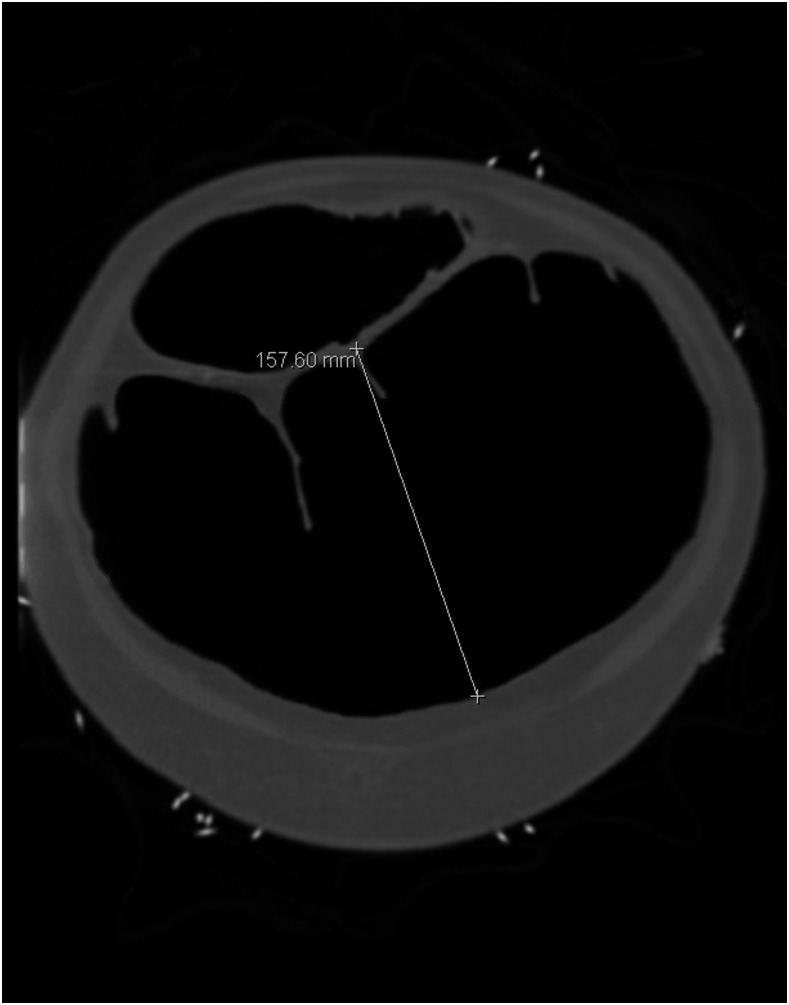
Computed tomographic study of the abdomen and pelvis in the coronal plane revealing a 16-cm dilatation of the transverse colon.
